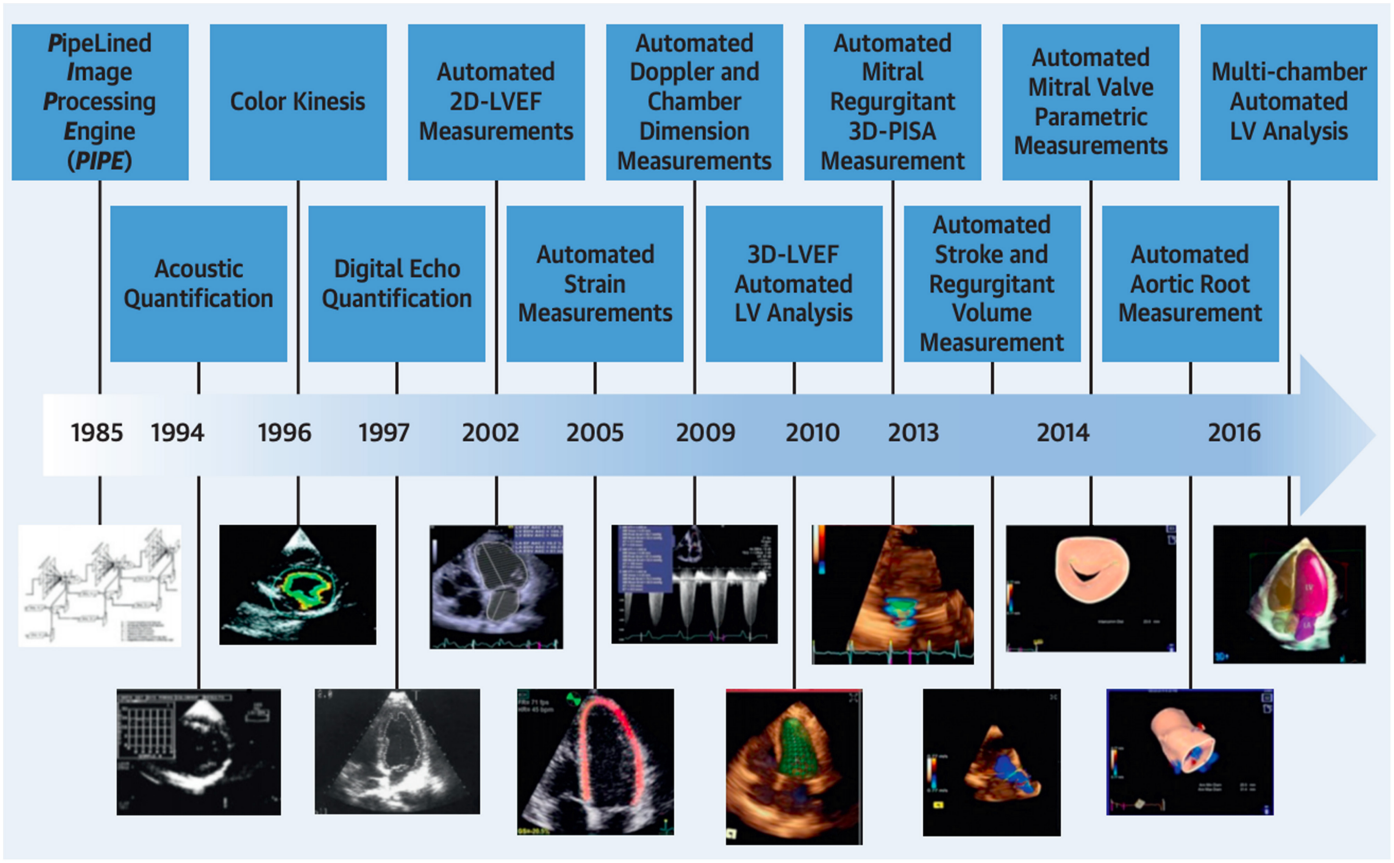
Temporal progression in automated quantification in echocardiography. Adapted from Nolan et al. (10). Permission obtained from the publisher.
